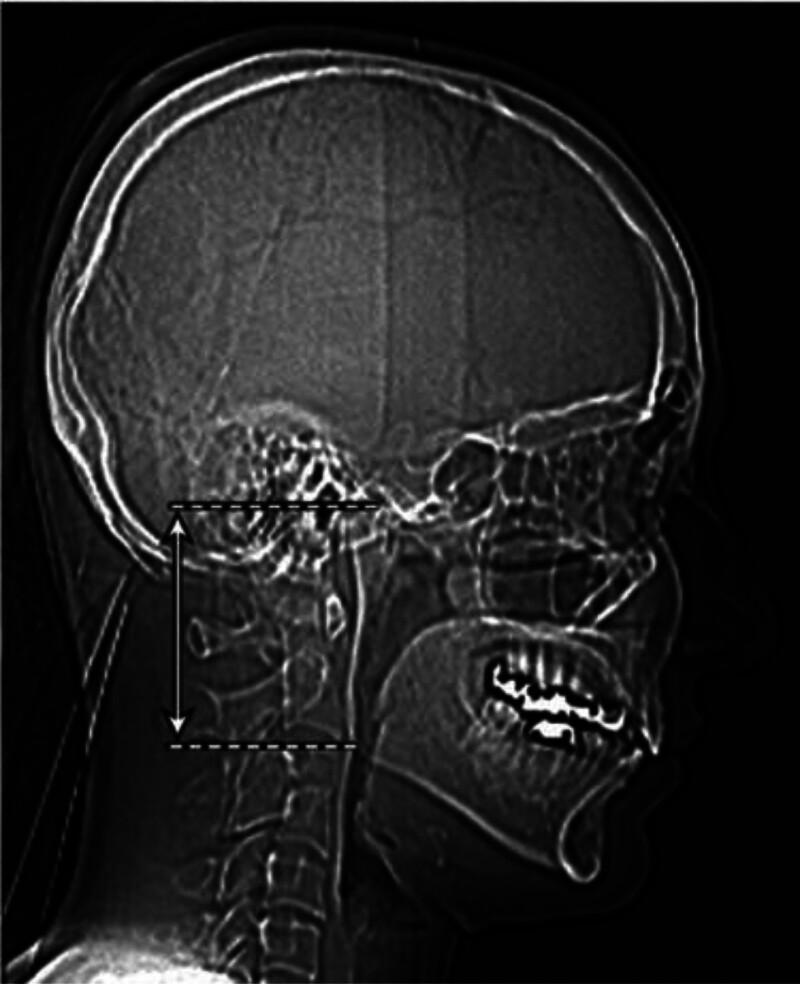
Demonstration of mandibular ramus length (MRL) measurement on a sagittal CT section. CT = computed tomography.
